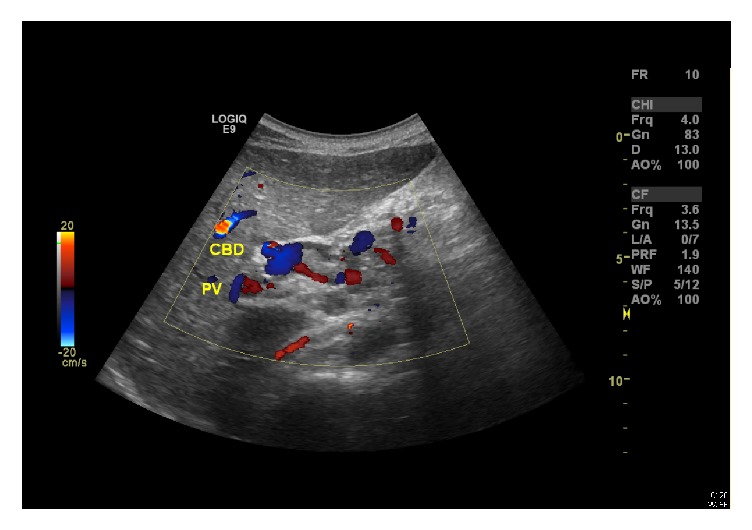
Abdominal ultrasound (post-ERCP with stenting) showing multiple intraluminal stones in the gallbladder with thick biliary sludge and diffusely thickened wall. Biliary stent is seen with the visualized portion of distal CBD that also appears slightly dilated at the porta hepatis. Minimal central intrahepatic biliary dilatation is also seen. Status after splenectomy.
